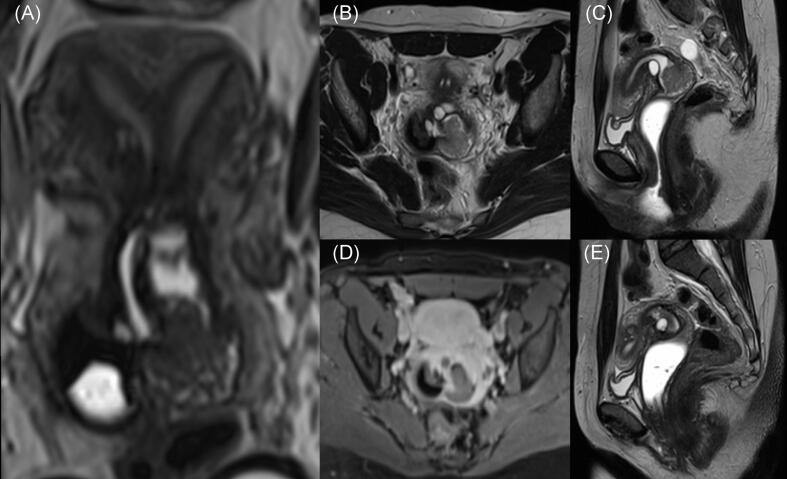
Magnetic resonance imaging of the abdomen. (A) 3D T2-weighted reconstructed image in the long axis showing a septate uterus with duplicated cervices and a longitudinal vaginal septum. The mass in the left cervix demonstrates intermediate T2 signal on axial (B) and sagittal (C) images and is hypovascular (D), consistent with cervical malignancy. (E) Sagittal T2-weighted image shows no suspicious lesions in the right cervix.
